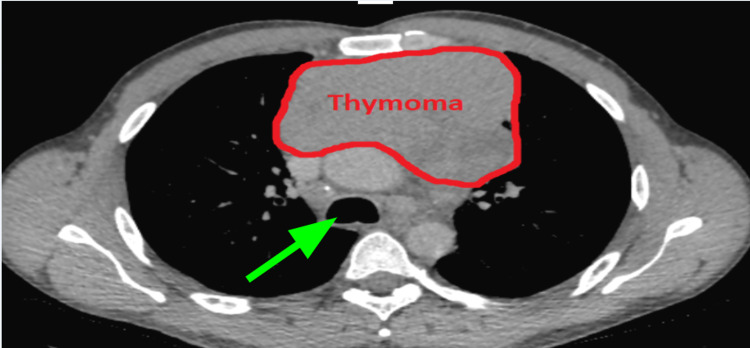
Axial view of 13.1 × 9.4 × 4.5-cm thymoma on computed tomography scan. Rightward deviation of the airway (green arrow) can be noted anterior to the spinal column.
